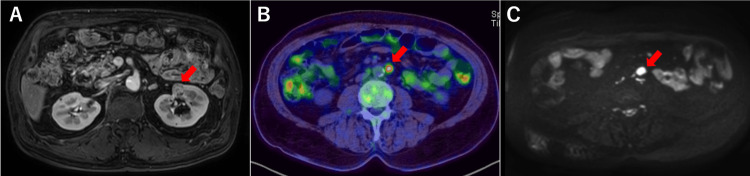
Imaging findings at presentation. A: Computed tomography (CT) shows 24 × 18 mm mass in the upper pole of the left kidney (arrow). B: 18F-fluorodeoxyglucose (FDG)-positron emission tomography/computed tomography reveals multiple enlarged para-aortic lymph nodes with increased FDG uptake (arrow), measuring up to 10 mm in the short axis diameter. C: Diffusion-weighted imaging (DWI) with apparent diffusion coefficient (ADC) mapping showed the restricted diffusion of the corresponding retroperitoneal lymph nodes (arrow).
